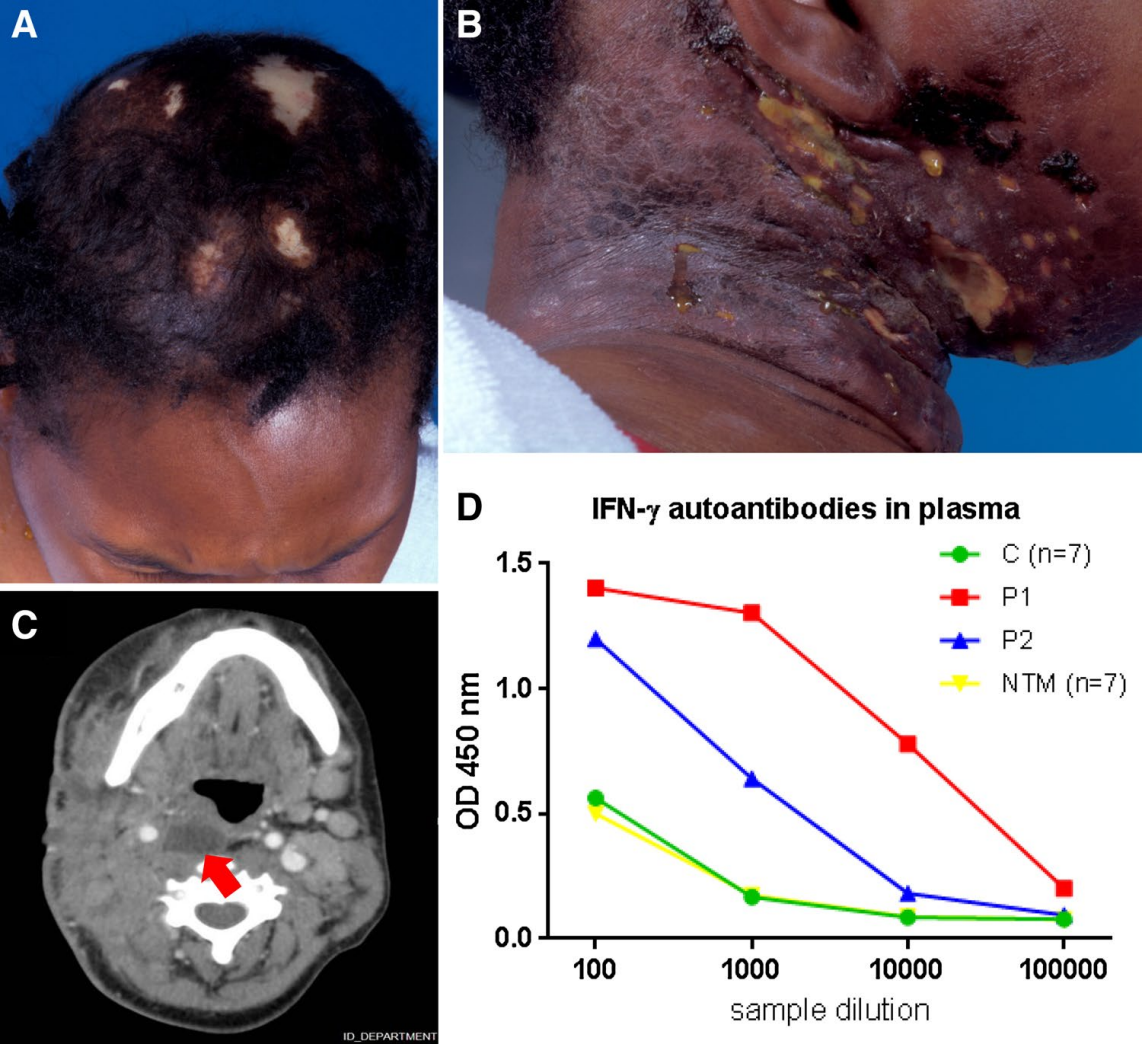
On clinical examination, alopecia (a) and multiple fistula of a necrotizing right-sided cervical mass (b) are noted. CT scanning of the neck (c) reveals an inflammatory mass from the mucosal space extending to the skin from the nasal pharynx all the way down to the upper thoracic aperture, with multiple abscesses including one located prevertebral (*arrow*). The right carotid artery and jugular vein are taken up in the mass, with the vein being compressed. *The left side* shows multiple enlarged lymph nodes. The graph (d) shows the presence of IFN-γ autoantibodies in plasma of seven healthy controls (C, mean), one patient with a high concentration of strongly inhibiting IFN-γ autoantibodies (P1), the current patient (P2), and seven patients with NTM infections (NTM, mean) as measured by ELISA. Plates were coated with IFN-γ and plasma was allowed to bind before detection of the anti-IFN-γ autoantibodies with HRP-labelled anti-IgG
